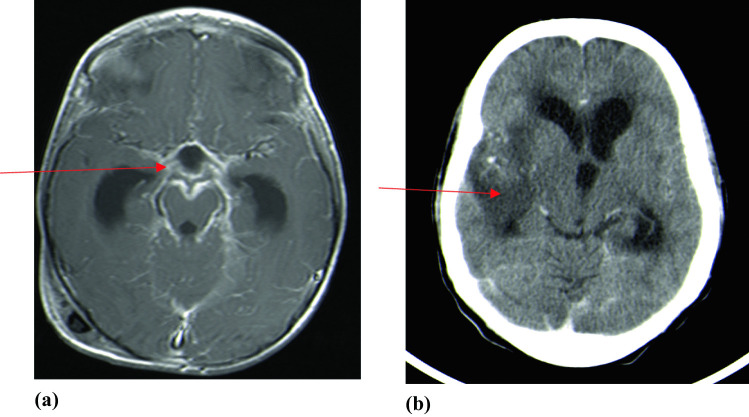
a T1-weighted post contrast MRI brain scan showing basal enhancement and b post contrast CT brain scan showing right middle cerebral artery territory infarct
